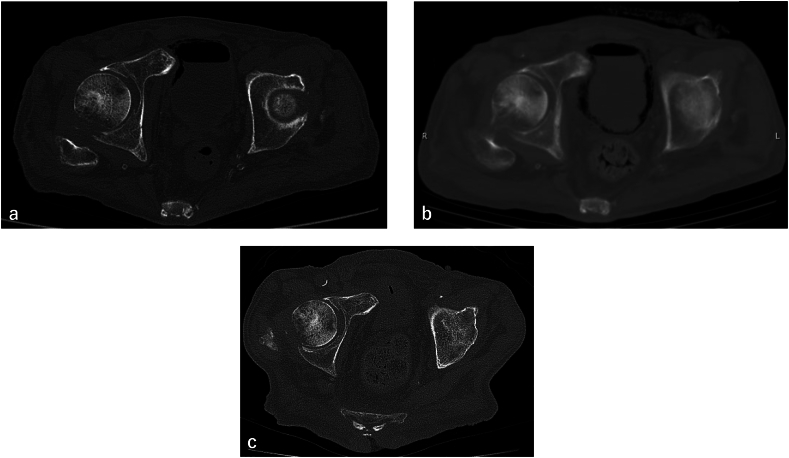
Serial computed tomography (CT) of the pubic nonunion fracture site and bladder (a) Emphysema of the bladder wall in contact with the pubic nonunion site is seen after starting weight bearing. (b) Emphysema has spread over the bladder wall in 2 weeks. (c) Emphysema has disappeared after 2 months.
